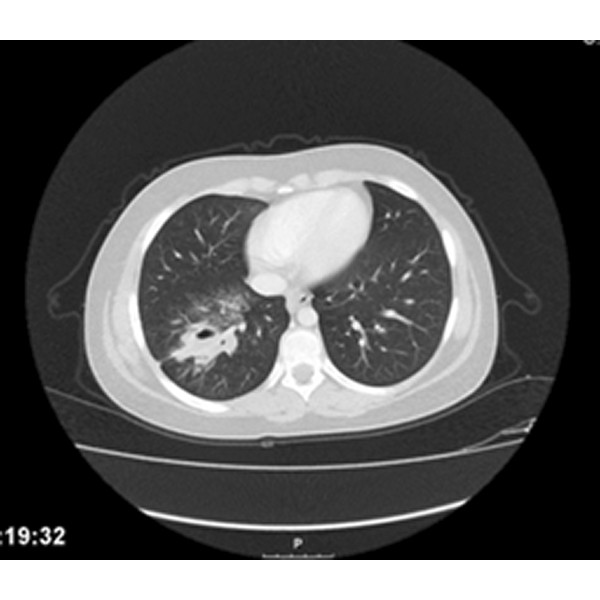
Cavitary lesion mimicking tuberculosis on chest computed tomography.
